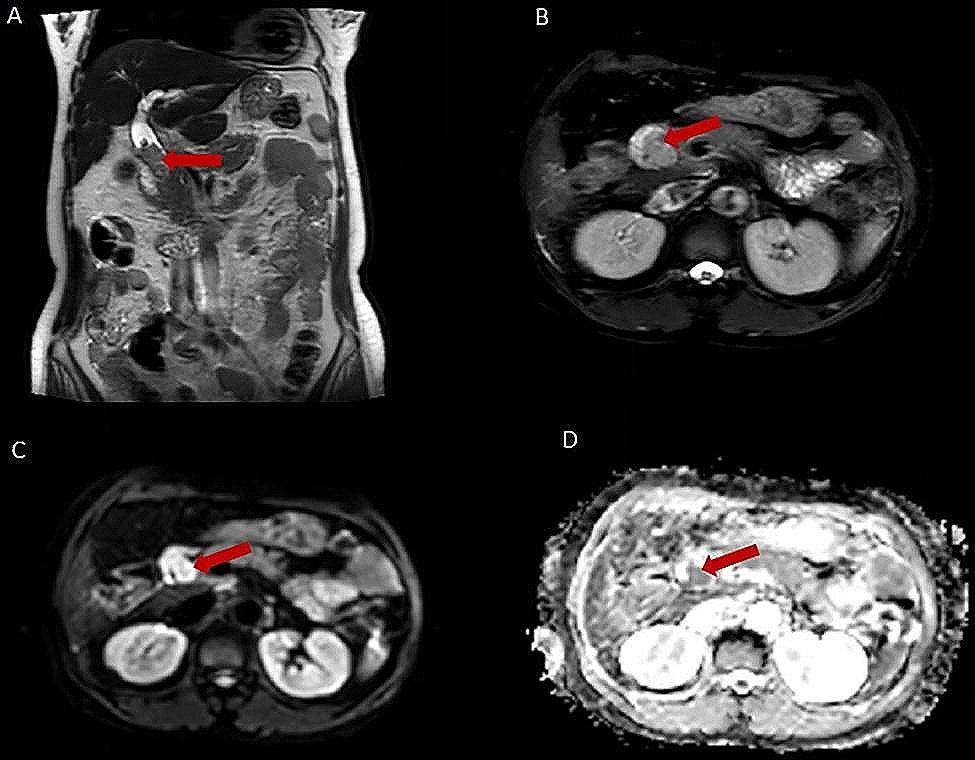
MRI image of a patient with distal cholangiocarcinoma. (A) and (B) T2-weighted images showing the lesion’s location, size, morphology, and MRI signal characteristics. (C) Diffusion-weighted images showing a intermediate/high signal for the tumor. (D) Apparent diffusion coefficient (ADC) map showing a low signal for the tumor. The red arrows pointed to the tumor
